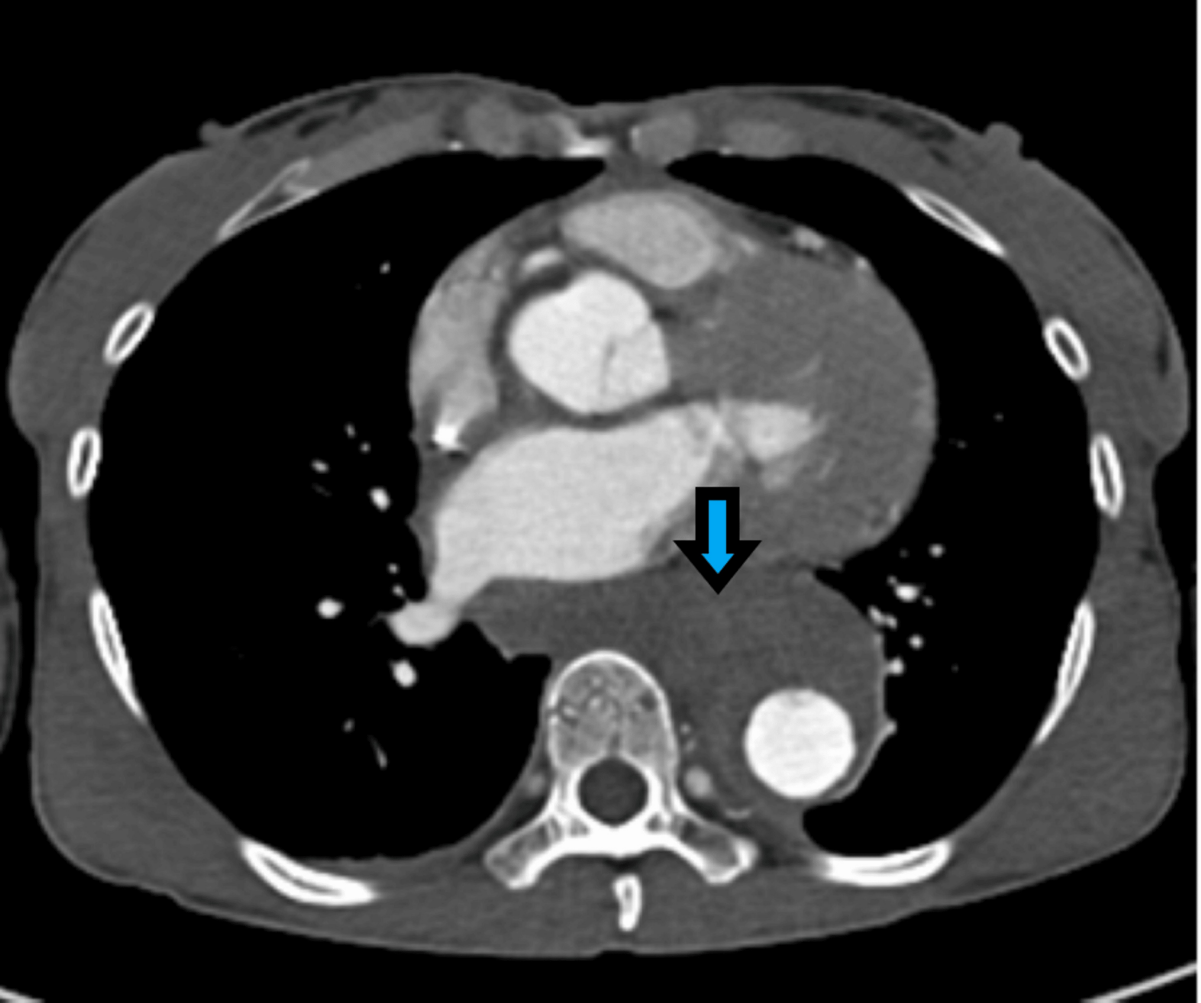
Computed tomography angiogram, axial plane, demonstrating a large hematoma within the false lumen of a type B aortic dissection
